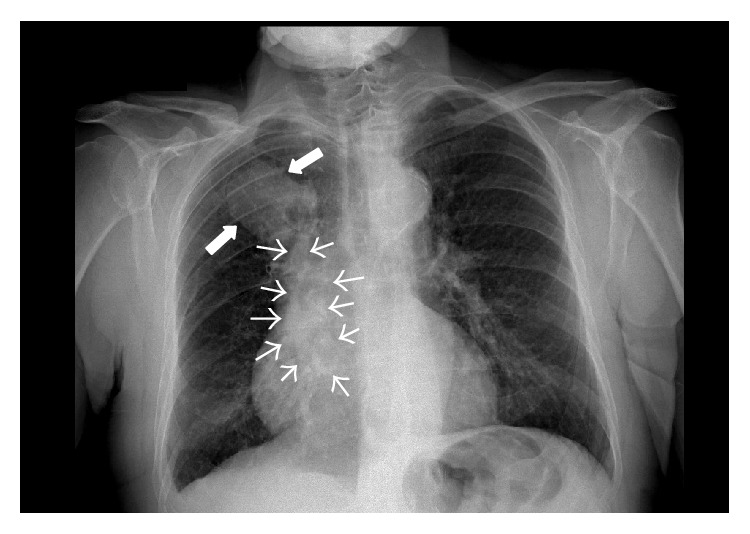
Chest X-ray showing a rounding mass projecting over the right upper lobe and extending towards the right upper portion of cardiac silhouette.
